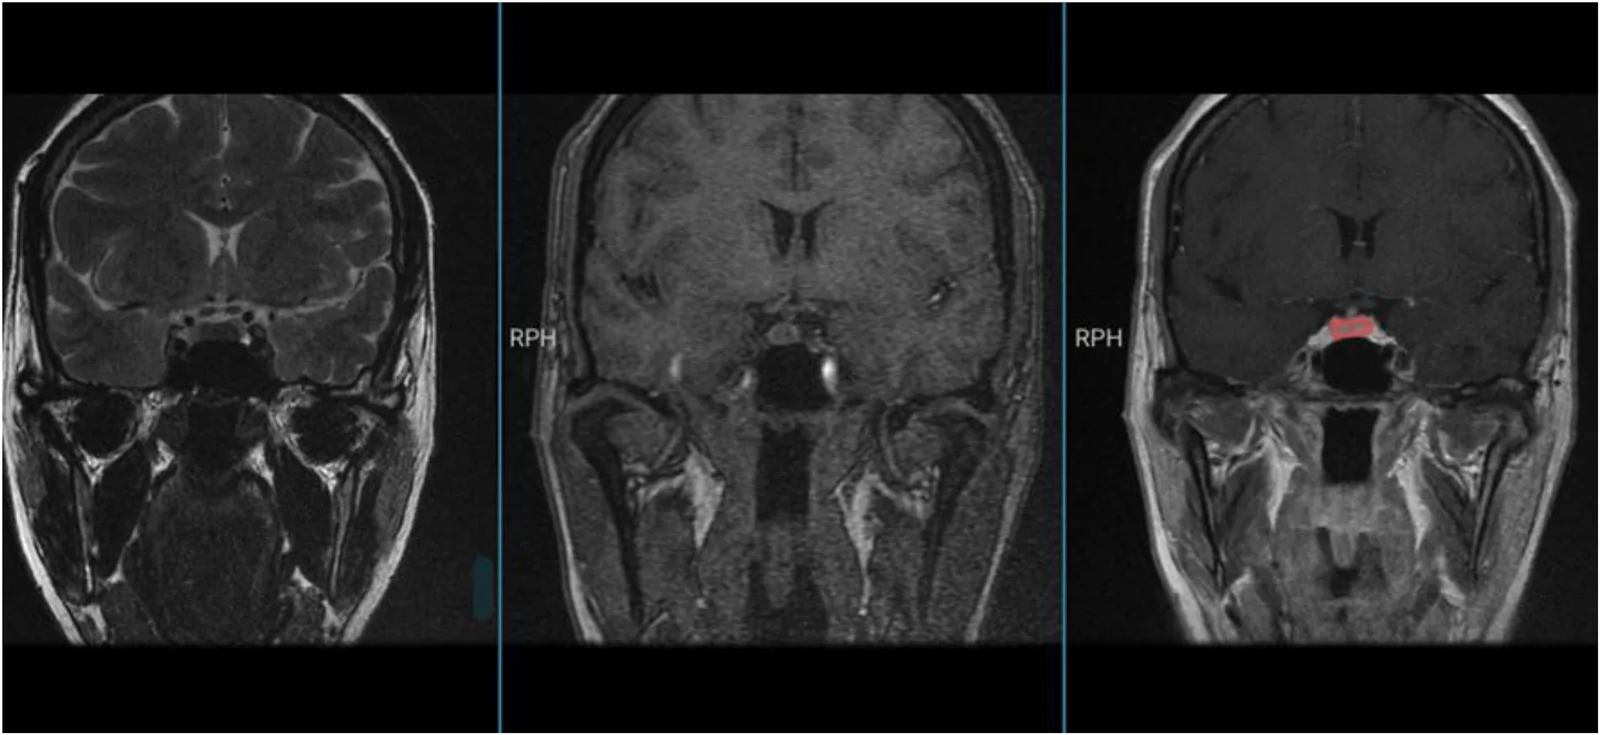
Magnetic resonance imaging of patient 2: flat pituitary adenoma with 1.1-cm largest diameter showing a hypointense signal obtained in the T2-weighted sequence.
